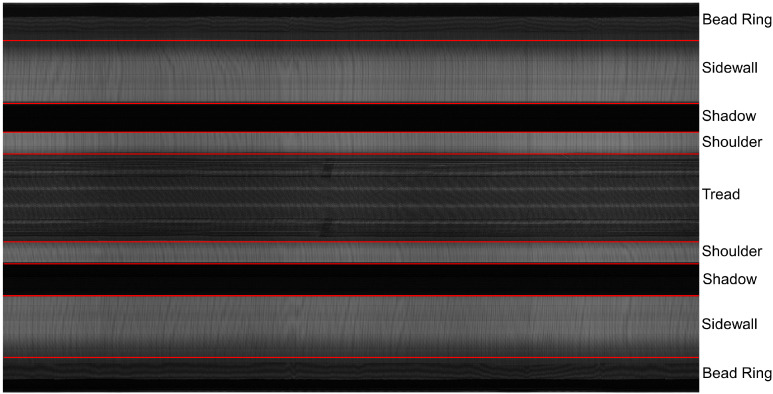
A horizontally displayed entire defect-free X-ray image, divided into five areas: bead ring, sidewall, shadow, shoulder and tread.
